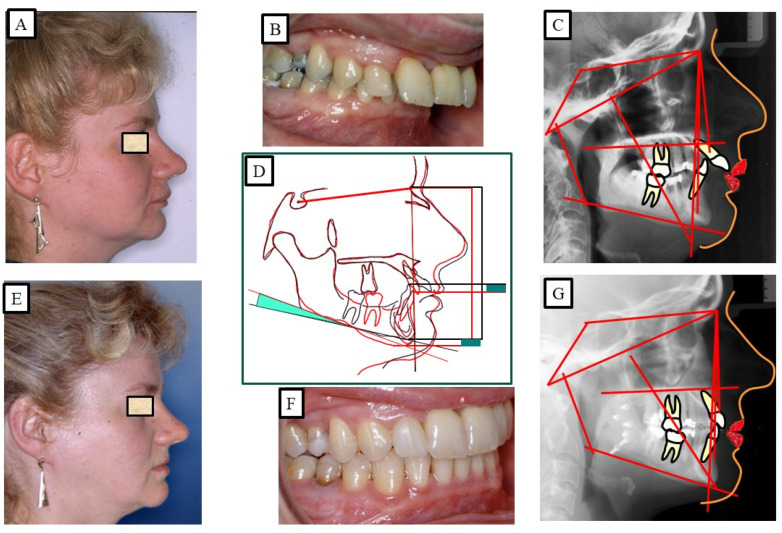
An adult patient with Class II dysgnathy and deep bite (skeletal and dentoalveolar). The correction of skeletal Class II and skeletal deep bite is performed via intrusion of the frontal teeth and combined orthodontic surgical treatment. Posterior rotation of the mandible during surgical mandibular advancement caused bite elevation and lengthening of the lower facial height. (A–C) show before orthodontic surgical treatment. (D) shows the over-positioning of the pre-treatment (black) and post-treatment (red) radiographs due to the changes in the sagittal and vertical dimensions. The surgical rotation of the mandible caused the opening of the mandibular angle, which led to the lengthening of the lower facial height. (E–G) show after orthodontic treatment.
